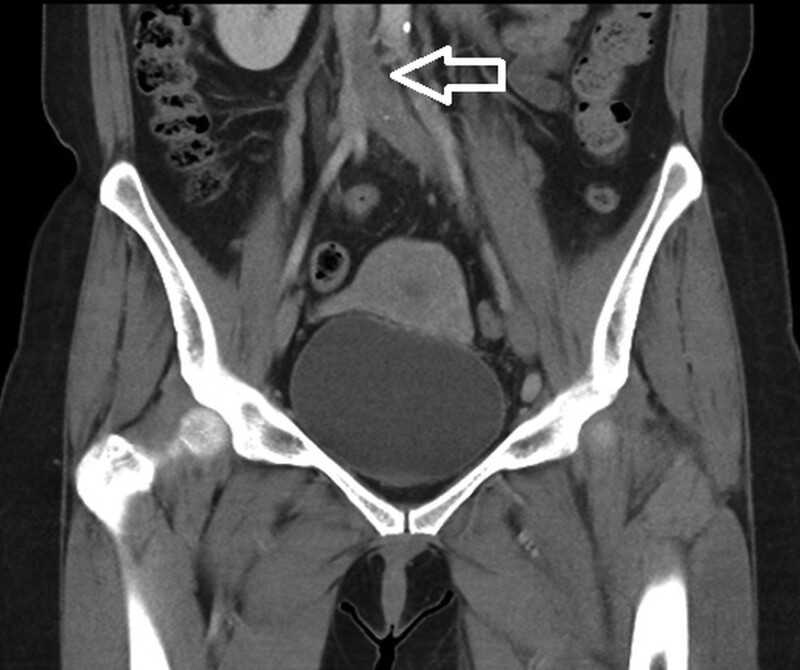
Coronal computed tomography indicating thrombosis (arrow) in the inferior vena cava and left common iliac vein.
